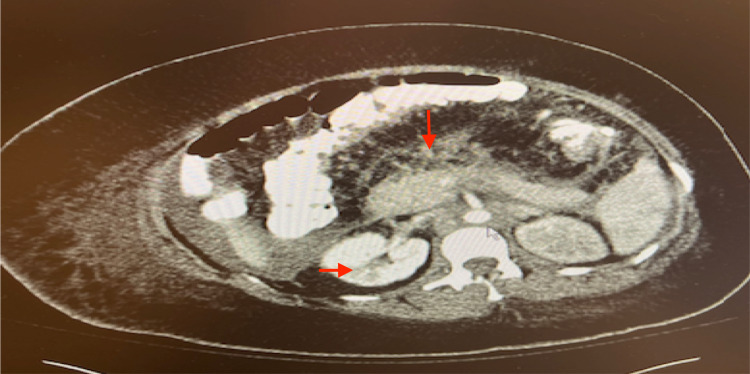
Pancreatitis and renal infarction on CT scan of abdomen/pelvis with IV contrast Computed Tomography (CT) showing severe left hydronephrosis without discrete ureteral calculus. There is also left renal cortical hypo-enhancement, which is suggestive of pyelonephritis and acute pancreatitis (vertical red arrow) but no pancreatic necrosis or peri-pancreatic fluid. Bilateral kidneys were significant for renal infarctions (horizontal arrow).
